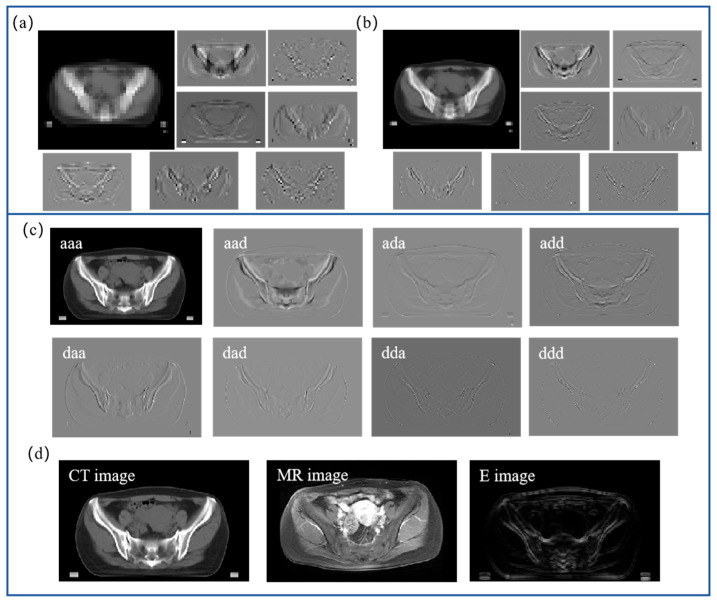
(a) In the third stage, eight sub-images are obtained after the decomposition of the CT image. (b) In the second stage, eight sub-images are obtained after the decomposition of the CT image. (c) In the first stage, eight sub-images are obtained after the decomposition of the CT image. (d) The patient’s raw CT and MR images and enhanced processing of high-frequency components.
